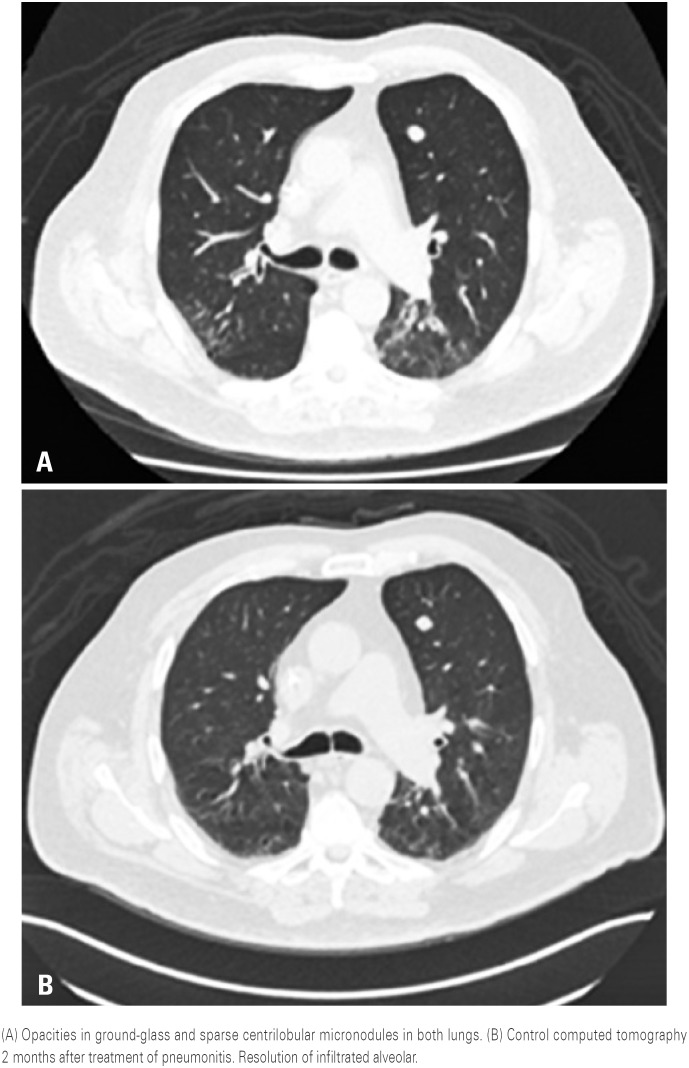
Computed tomography scan of a patient with immunotherapy-induced pneumonitis
